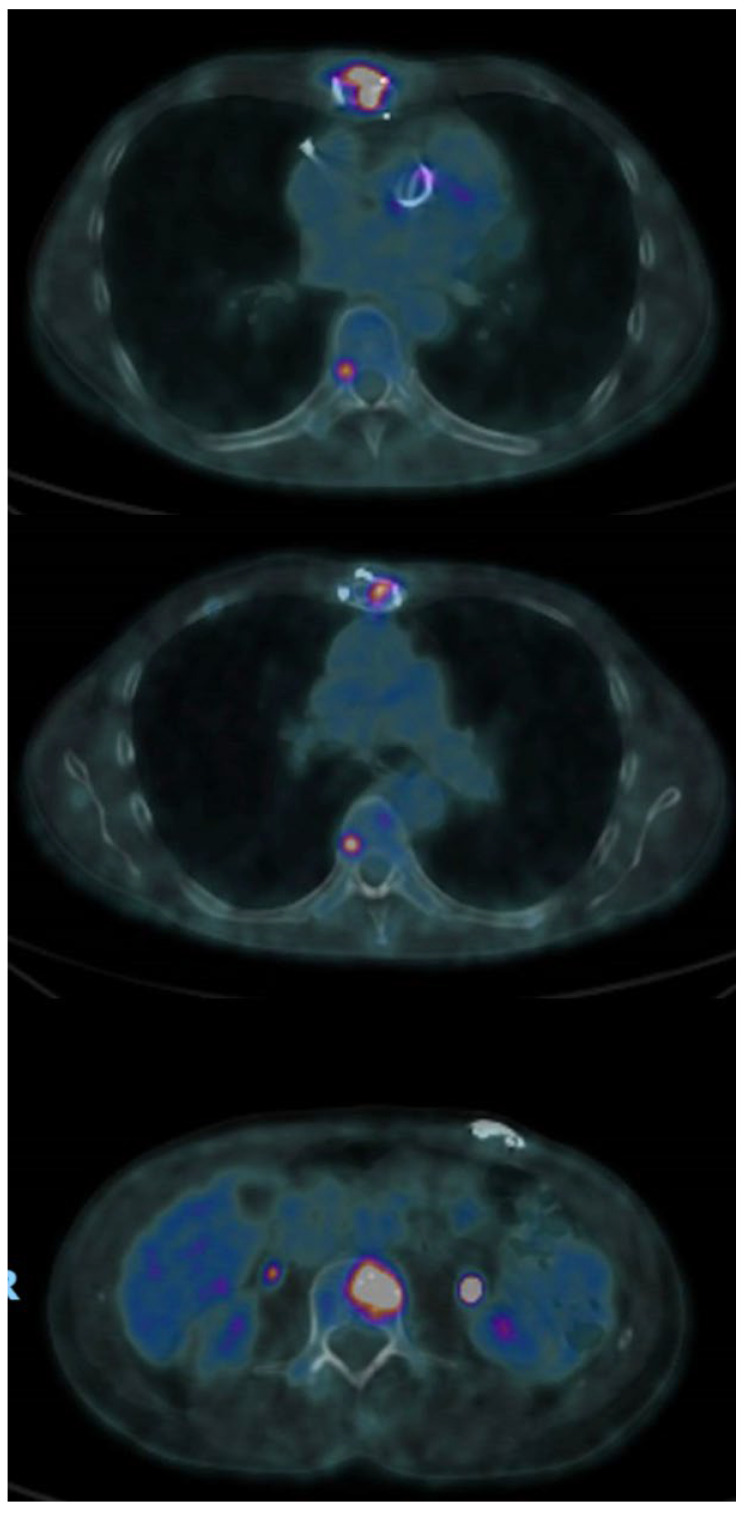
Multiple myeloma—CT PET image shows multiple focal areas of vertebral bone marrow with increased metabolic activity at T6 (above), T10 (middle), L1 (below).
